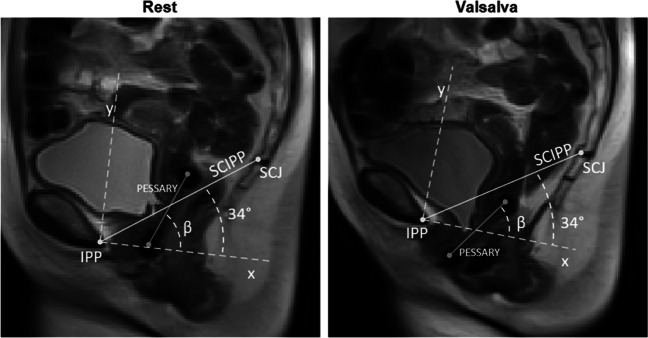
Landmarks on dynamic magnetic resonance imaging showing the pessary angle. The *solid dark grey* represents the pessary and is drawn through the pessary cross-sectional points. The Pelvic Inclination Correction System (*PICS*) line is defined as a line 34° clockwise from the sacro-coccygeal inferior pubic point (*SCIPP*) line (*solid light grey*): a line from the inferior pubic point (*IPP*) to the sacrococcygeal junction (*SCJ*). The origin is located at IPP. The PICS is identical to the x-axis, pointing posteriorly. The y-axis points cranially, perpendicular to the x-axis through the IPP. The definition of the pessary angle (*β*) is the angle between the line through the pessary cross-sectional points (*solid grey*) and the PICS line (*x*)
